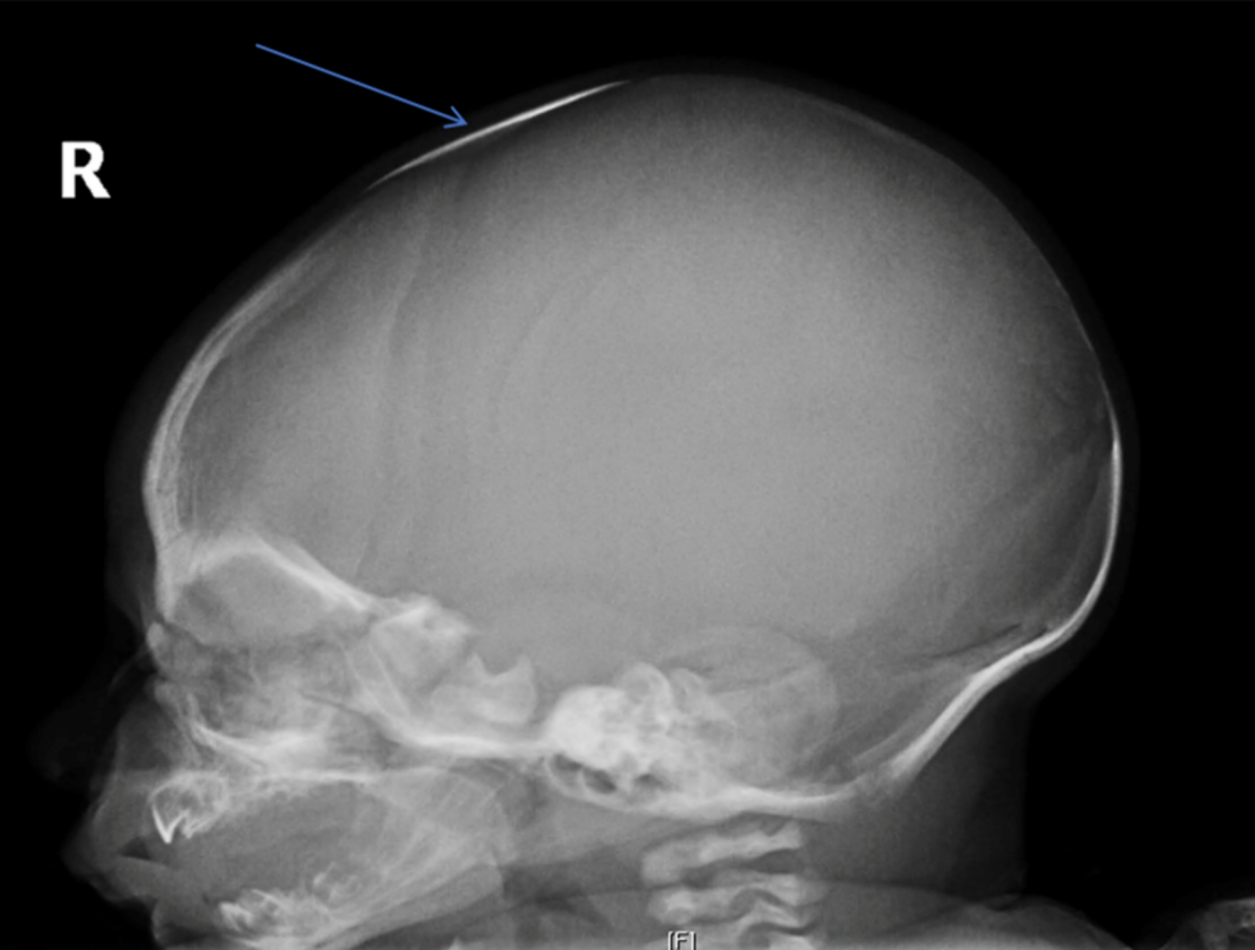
Left lateral view from the cranial X-ray series Blue arrow depicts the presence of Wormian bone in the anterior fontanelle.
